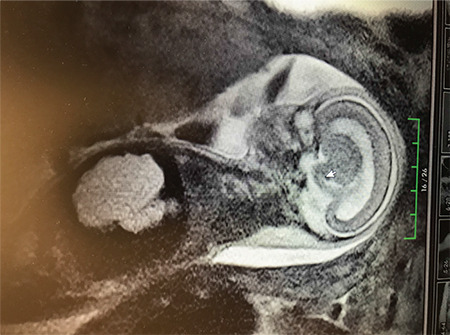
Magnetic resonance imaging of agenesis of corpus callosum
